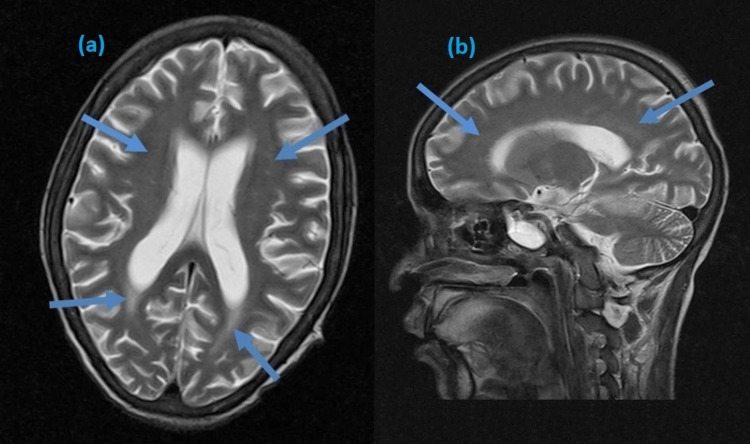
Brain MRI showing hyperintense signals in the temporal and parietal regions in axial (a) and sagittal (b) planes
